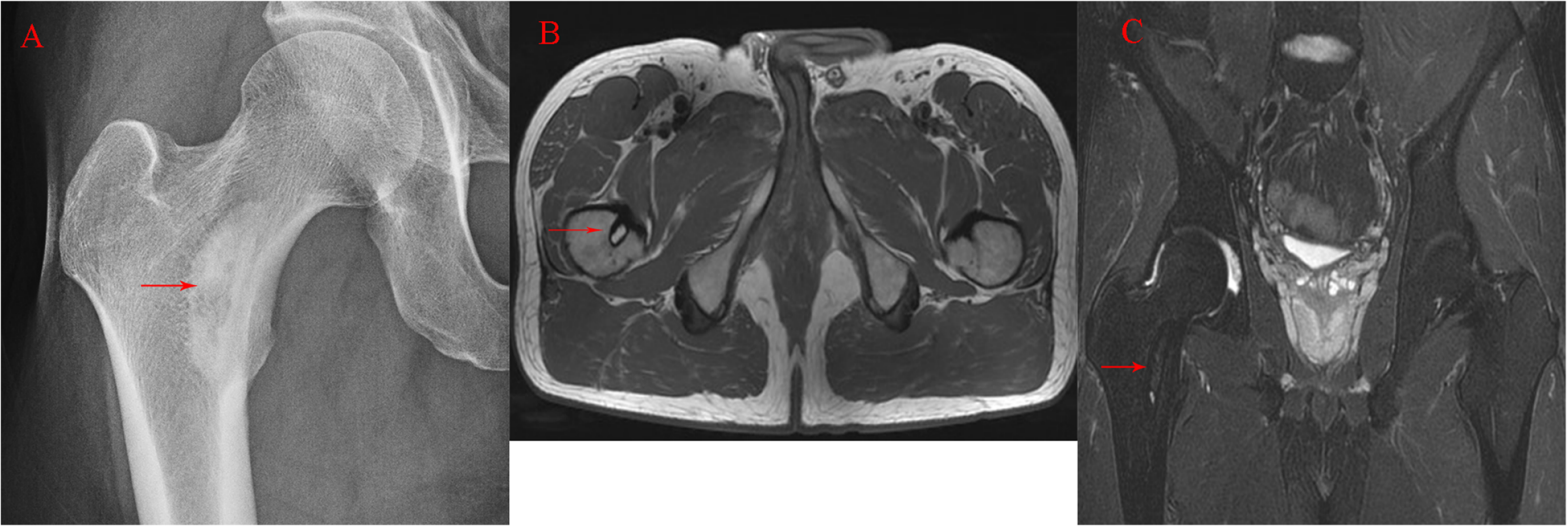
a An enchondroma is located in the calcar femorale in the coronal-view plain radiography (red arrow) b c It is also can be seen in a transverse plane of T1 weighted magnetic resonance image and the coronal plane of T2 weighted magnetic resonance image respectively
